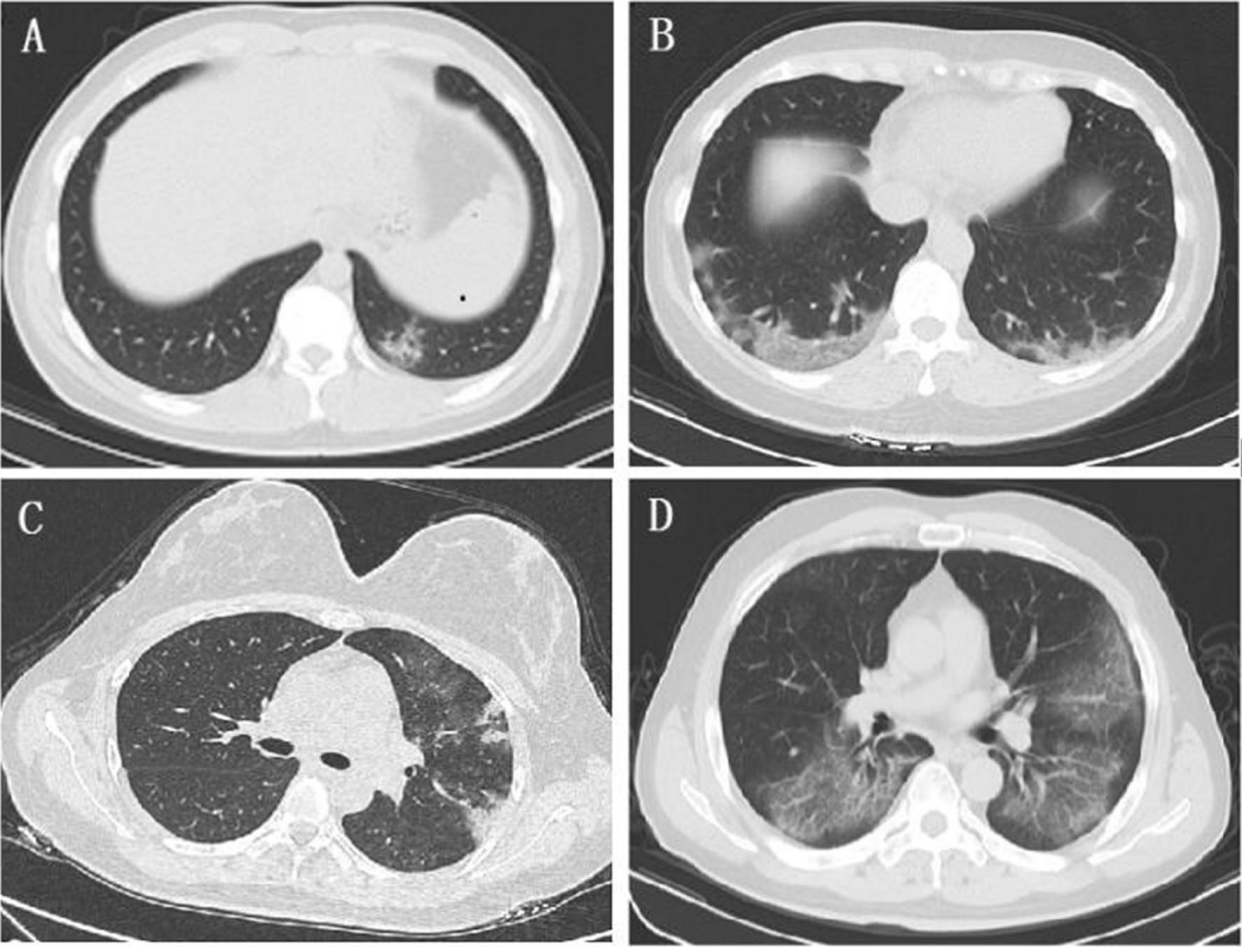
Chest CT images of COVID-19 cases. GGO, patchy ground-glass opacity; RR, respiratory rate; BP, blood pressure. A Chest CT image of a patient from The United Kingdom on the 1st day of hospitalization showing patchy ground-glass opacity (GGO) in the subpleural area of the left lower lobe (dry cough, no dyspnoea, no supplementary O_2_ required, SpO_2_ 98%, RR 18 times/min, BP 151/66 mmHg). B Chest CT image of a patient from America on the 1st day of hospitalization showing multiple patchy and spherical GGOs in the lower lobe of the bilateral lungs with interlobular septal thickening (fever, sore throat, no dyspnoea, no supplementary O_2_ required, SpO_2_ 96%, RR 20 times/min, BP 110/70 mmHg). C Chest CT image of a patient from The United Kingdom on the 1st day of hospitalization showing multiple patchy consolidations and GGOs in the left lung (fever, dry cough, no dyspnoea, no supplementary O_2_ required, SpO_2_ 97%, RR 18 times/min, BP 124/67 mmHg). D Chest CT image of a patient from Spain on the 1st day of hospitalization showing multiple patchy GGOs and early consolidation in the bilateral lungs (fever, dyspnoea, supplementary O_2_ required 2 L/min by nasal cannula, SpO_2_ 97%, RR 21 times/min, BP 127/86 mmHg)
